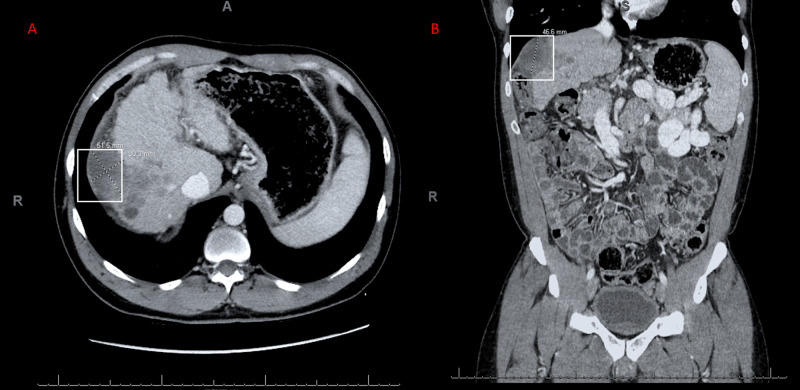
Growth of Exophytic Hepatic Mass Seen In CT of the Abdomen with Contrast One month after the initial diagnosis for HCC (hepatocellular carcinoma), CT (computerized tomography) of the abdomen with contrast demonstrated a growth in the exophytic mass. The mass measured 5.15 by 3.83 mm in the transverse view (Panel A) and 46.6 mm in the coronal view (Panel B).
